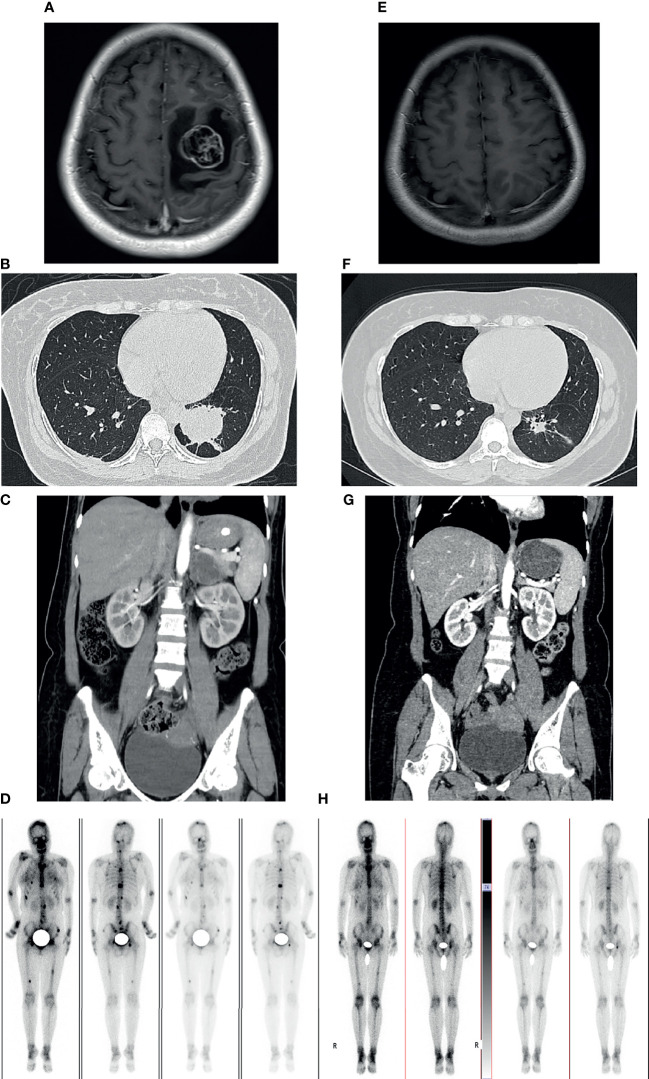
Imaging examinations before craniotomy and alectinib treatment (A–D). 10-months follow-up radiological evaluation (E–H). (A) Contrast-enhanced cranial MRI showed the lesion in the left frontal lobe. (B) CT scan of the chest showing the mass in left lower lobe of the lung. (C) CT enhancement scan showing the mass in the left adrenal. (D) 99mTc-MDP bone scintigraphy demonstrating multiple lesions of increased activity in spine, pelvis, skull, ribs, skull and femurs. (E–H) Radiological evaluation demonstrating a dramatical shrinkage of all known lesions after 10 months.
